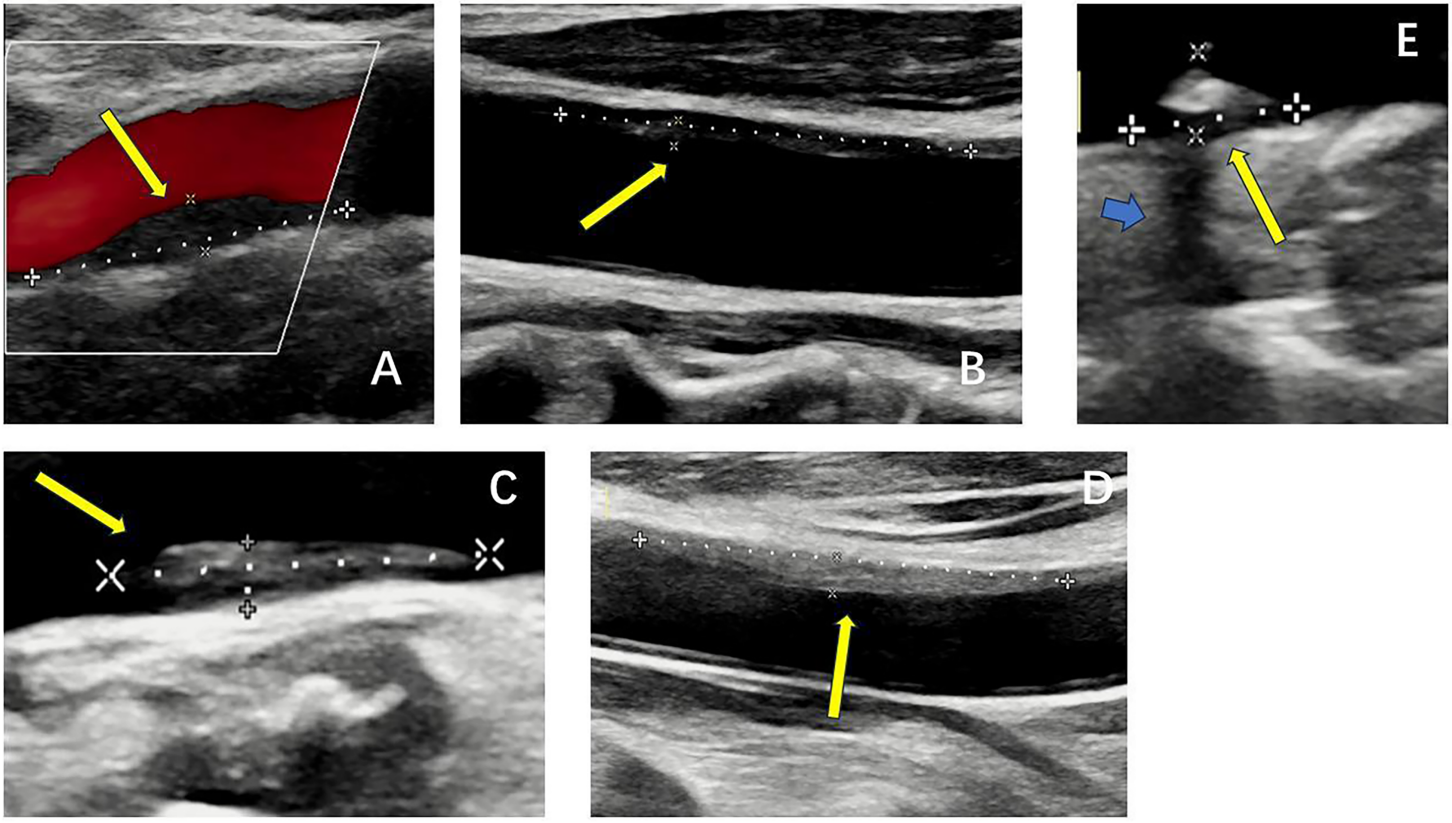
B-mode ultrasound images of five types of plaques distinguished by the gray-weale–nicolaides (GWN) classification including (A) uniformly echolucent plaque, (B) predominately echolucent plaque, (C) predominantly echogenic plaque, (D) uniformly echogenic plaque, and (E) heavy calcification. The yellow arrows indicate plaques. The blue arrow indicates the shadow.
